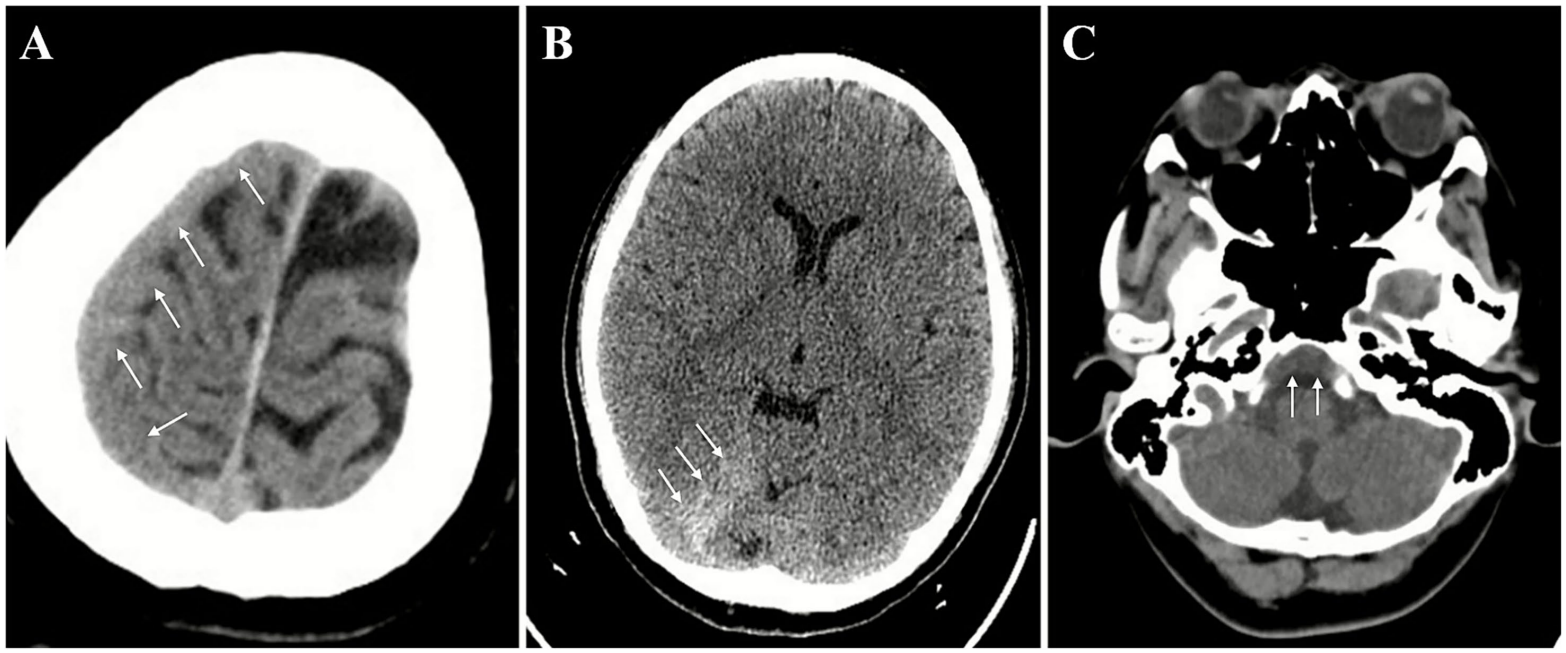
Initial cranial CT imaging upon admission. Subdural hematomas (arrows) were indicated in the right frontal parietal lobe (A), right cerebellar tentorium (B), and above the clivus (C).
